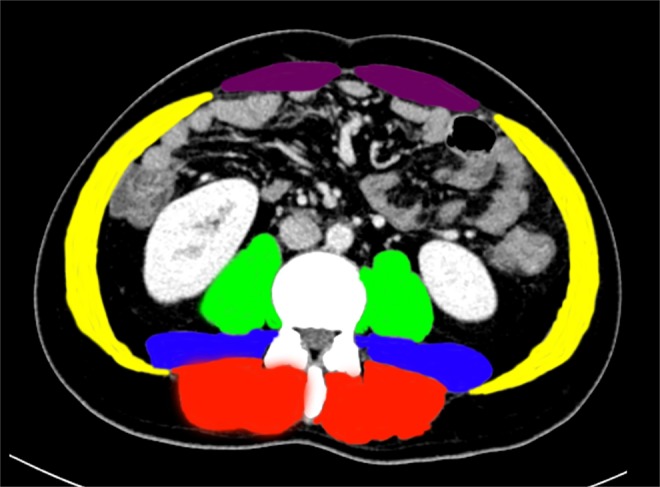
CT scan image analysis method of L3SMIl. Notes: Green is the psoas major, blue is the quadratus lumborum, red is the erector spinae, gold is the transversus abdominis muscle, internal and external oblique muscle, and purple is the rectus abdominis. Abbreviations: L3SMI, L3 skeletal muscle mass index.
